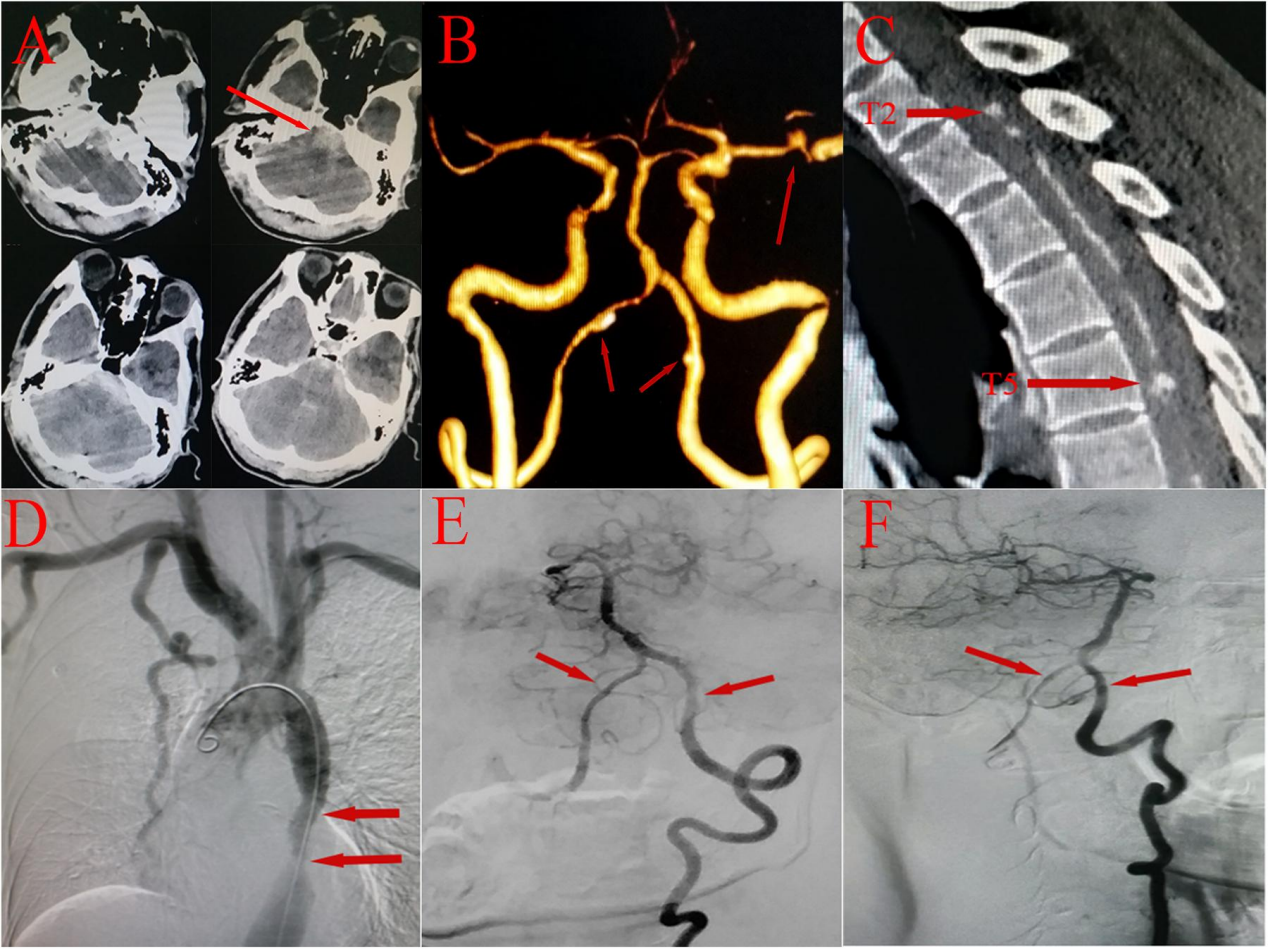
(A) CT scan of the skull base showing a high-density shadow around the foramen magnum and ambient cistern (red arrow). (B) Head CTA image demonstrating a left MCA aneurysm approximately 3 mm*2 mm in size and wall irregularities in the V4 segments of both vertebral arteries (red arrows). (C) Thoracic CTA image revealing multilevel circular enhancement lesions in the area of the anterior spinal artery (red arrows). (D) Aortic arch angiography revealing severe congenital descending aorta coarctation (red arrows). (E,F) Anteroposterior and lateral DSA showing no significant abnormalities in the V4 segments of either vertebral artery (red arrows).
